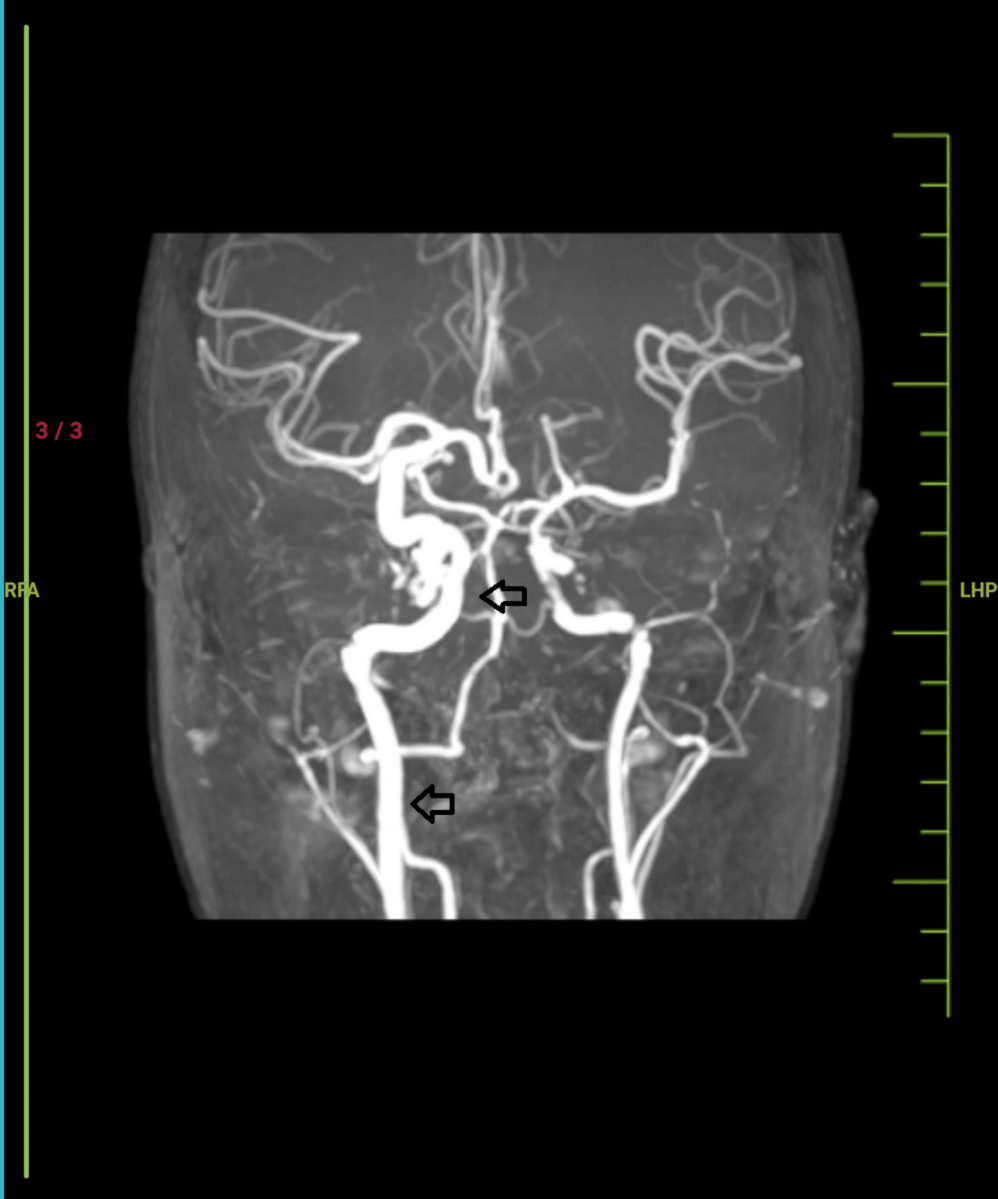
The coronal section of the MRI brain with MR angiography showing the dilated M1 segment of the right internal carotid artery (black arrows).
